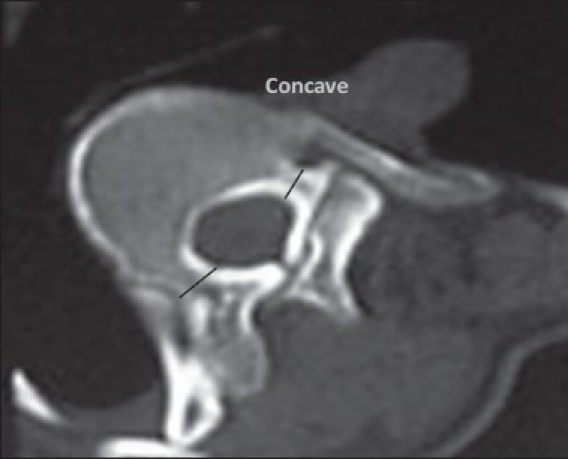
Axial CT image depicting transverse pedicle width
